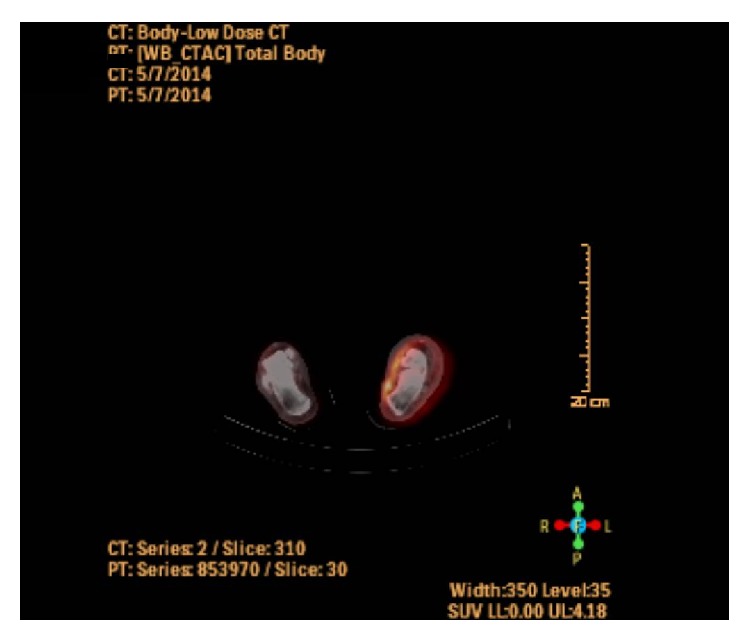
FDG-PET and CT scan showing heterogeneous uptake in the medial and lateral foot with a more focal uptake in the medial foot, anterior to the Achilles tendon.
